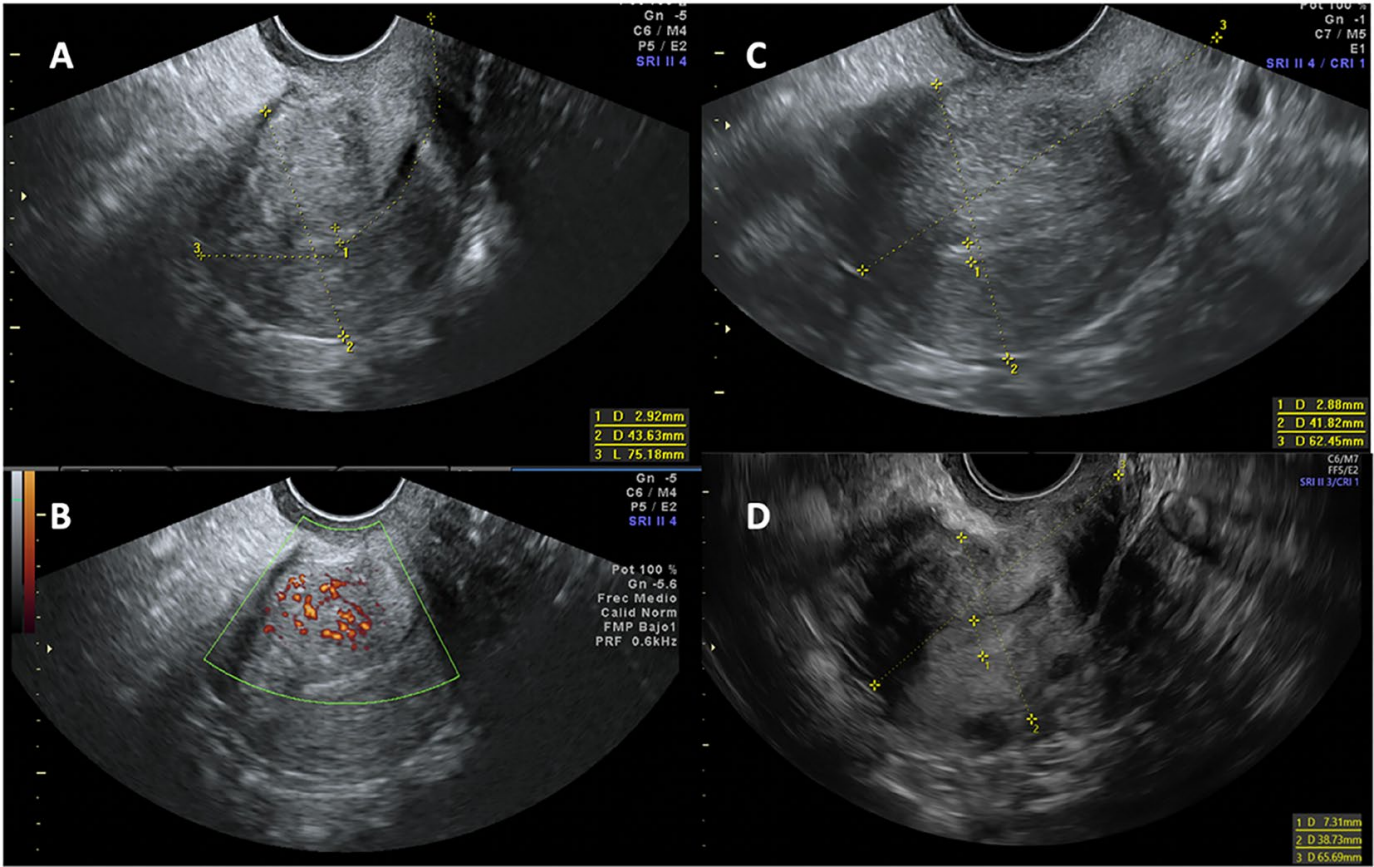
Adenomyosis sonographic evolution at baseline and at 12 and 24 months of follow-up. (A and B) Baseline ultrasound with the presence of 5 adenomyosis criteria; (A) Hyperechoic islands, fan-shaped shadowing, uterine wall asymmetrical thickening, interrupted junctional zone and (B) Translesional vascularity; (C) 12-month follow-up with hyperechoic islands and uterine wall asymmetrical thickening as mild signs of adenomyosis. (D) 24-month follow-up with no signs of adenomyosis.
